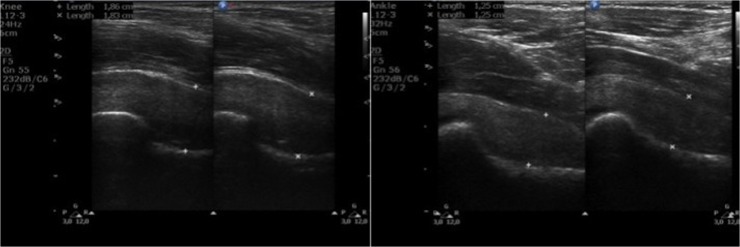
Ultrasound images of hip joints. (Left) Longitudinal scan of hip joint in a 32-year-old patient with an attenuated phenotype of MPS II. (Right) Longitudinal scan of hip joint in a 10-year-old patient with a severe phenotype of MPS II.
